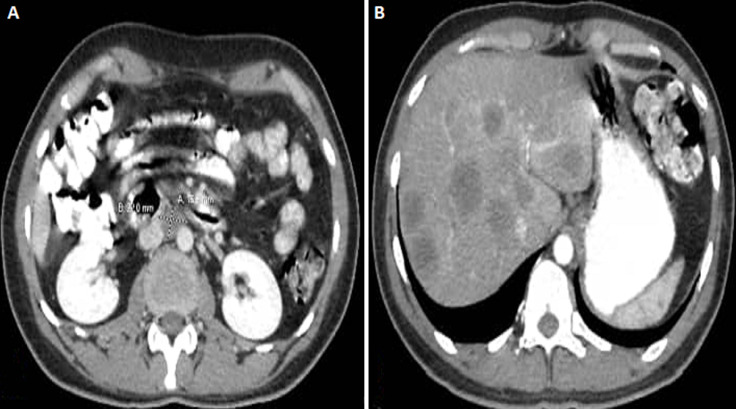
abdominal pelvic computed tomography scan showing: A) colonic mass; B) the liver metastasis
